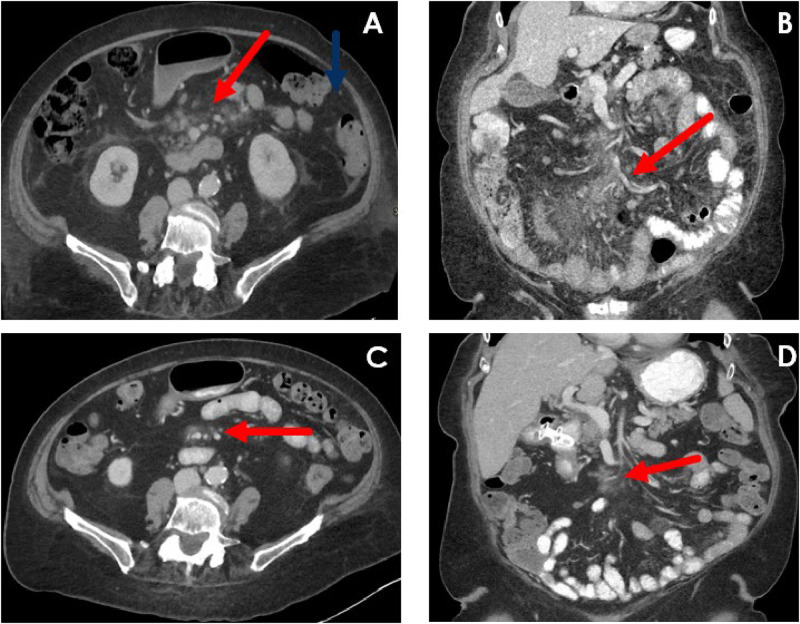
(A) Contrast-enhanced axial CT of the abdomen and pelvis demonstrates increased central mesenteric attenuation (“misty mesentery”), mesenteric lymphadenopathy (red arrow), as well as mesenteric soft tissue edema/infiltrate (blue arrow). The central mesentery involvement can be best appreciated on the coronal reformat (B, red arrow). After initiation of therapy, there is substantial decrease in the degree of mesenteric involvement with notable decrease in size and conspicuity of mesenteric lymph nodes (C, red arrow). The overall extent of mesenteric improvement is best appreciated on the coronal plane (D, red arrow). Findings are consistent with treatment effect.
